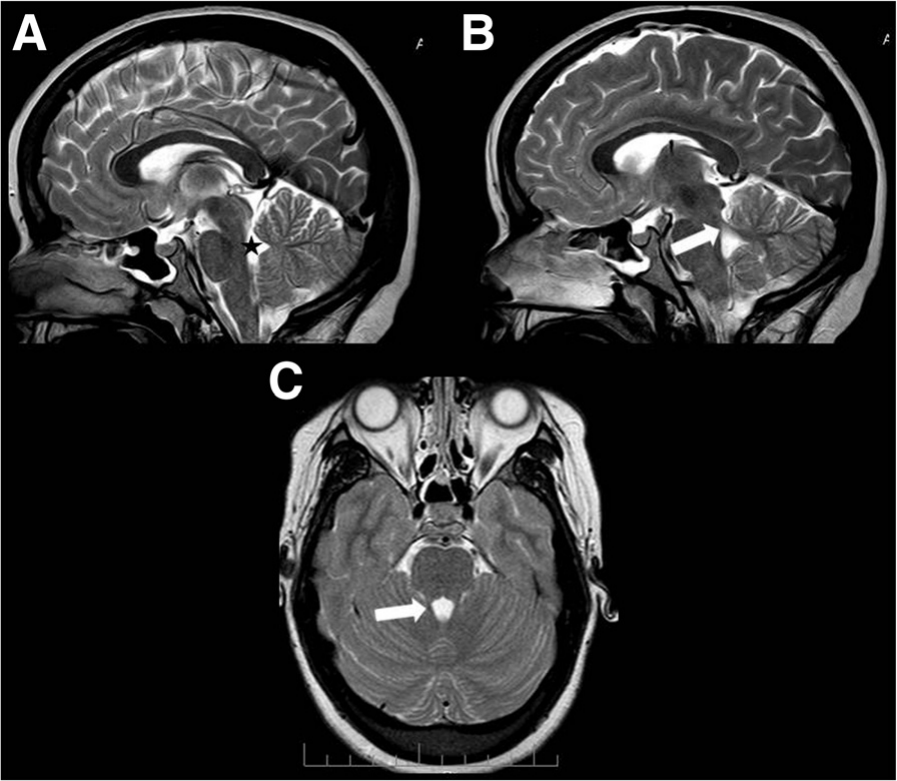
Normal MRI in a 19-year-old patient with COMA. a, b Sagittal and (c) axial T2-weighted MRI of patient #18 at age 19 years. a Midsagittal slice shows normal position of fastigium (star) and well developed vermis. b Parasagittal slice displays thin superior cerebellar peduncles (SCP) in oblique position (arrow). c Axial slice shows normal SCP (arrow). This MRI is normal
